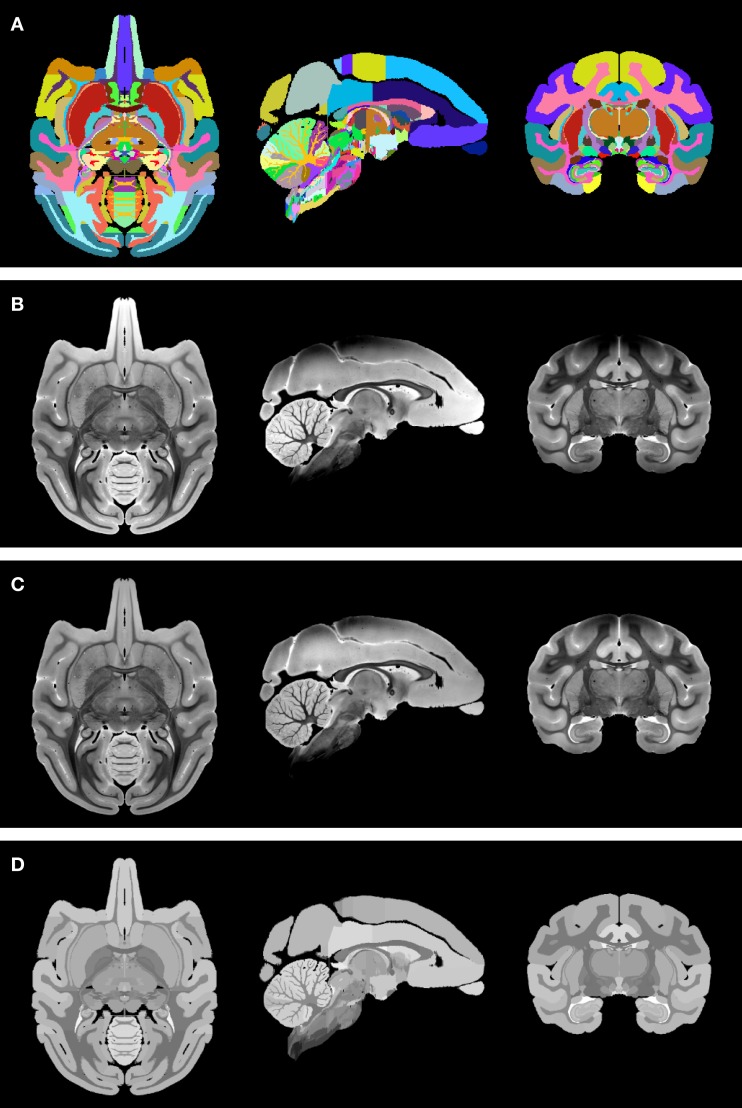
Preparation of the NeuroMaps label map and MR image: (A) symmetric, whole-brain label map. The two hemispheres use distinct labels, but left and right copy of each region are shown here using the same color for simplicity. (B) Symmetric, whole-brain MR image as provided through the NeuroMaps website. (C) Intensity bias-corrected MR image. (D) Region-averaged MR image. See text for details.
